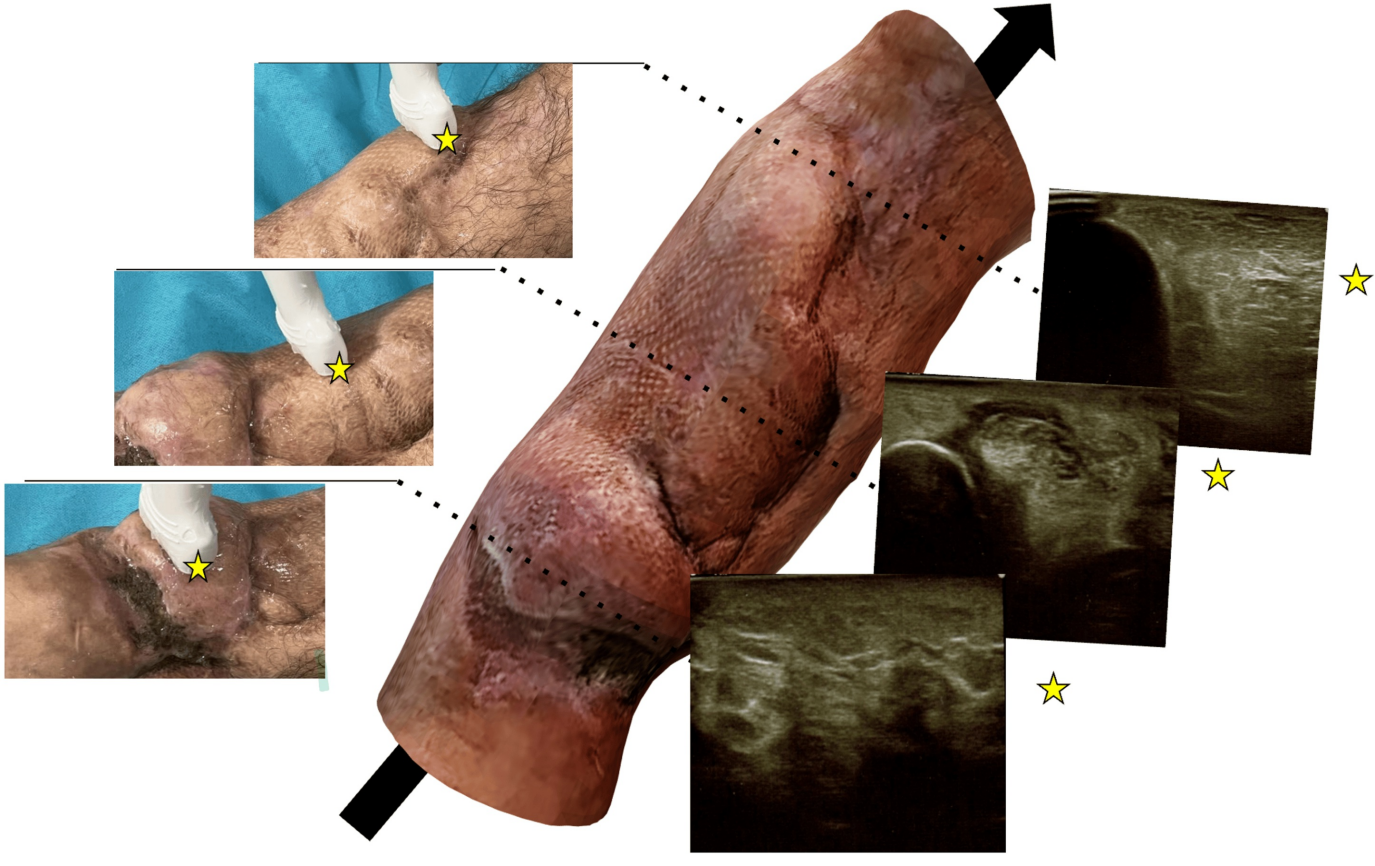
Summary of performed ultrasound imaging superimposed on a three-dimensional (3D) scan of the treated lower limb during a 33-month follow-up Doppler ultrasound in the flap pedicle. Visible retrograde flow spectrum
